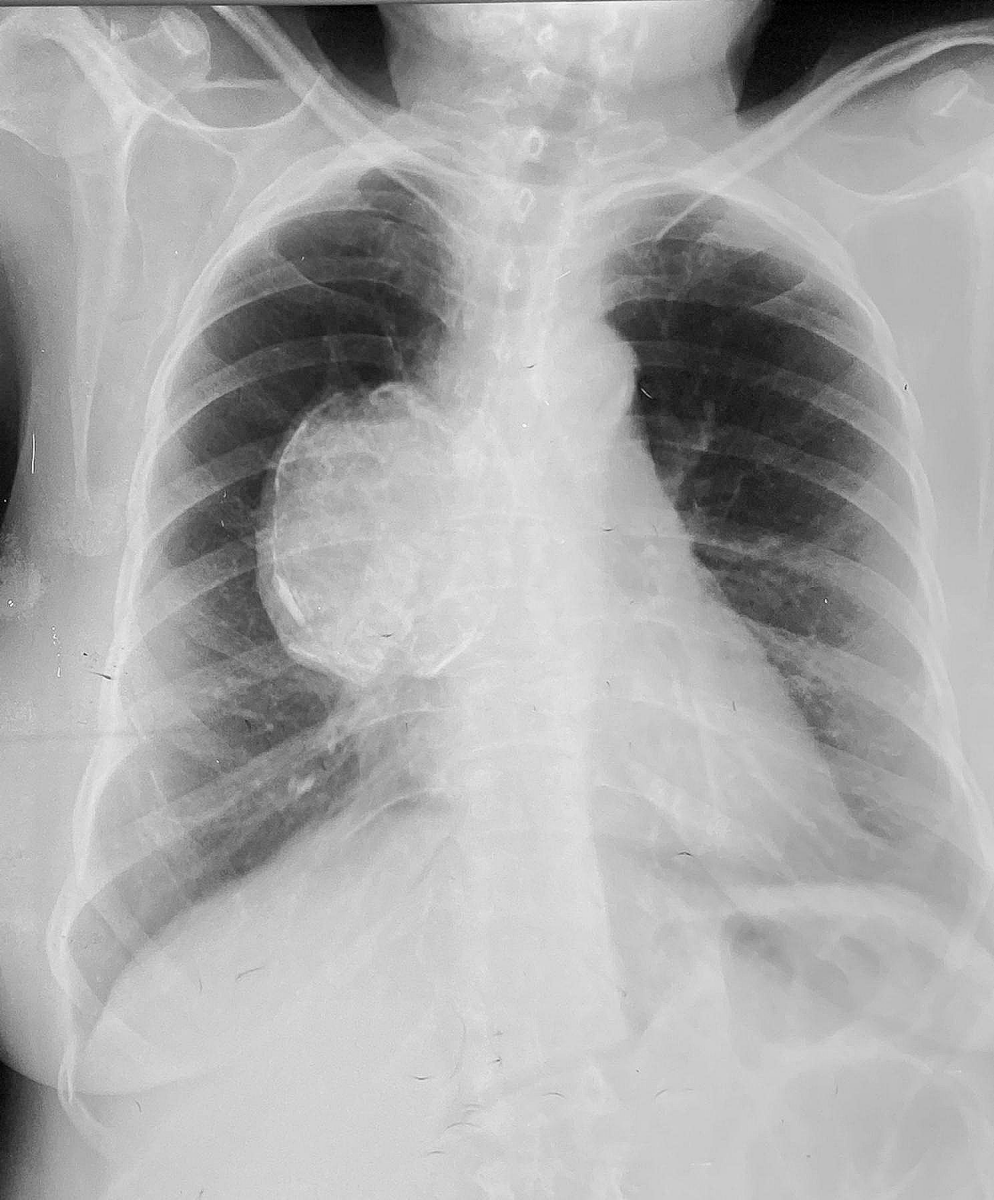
Preoperative chest Xray showing a round well limited opacity, with calcified limits
